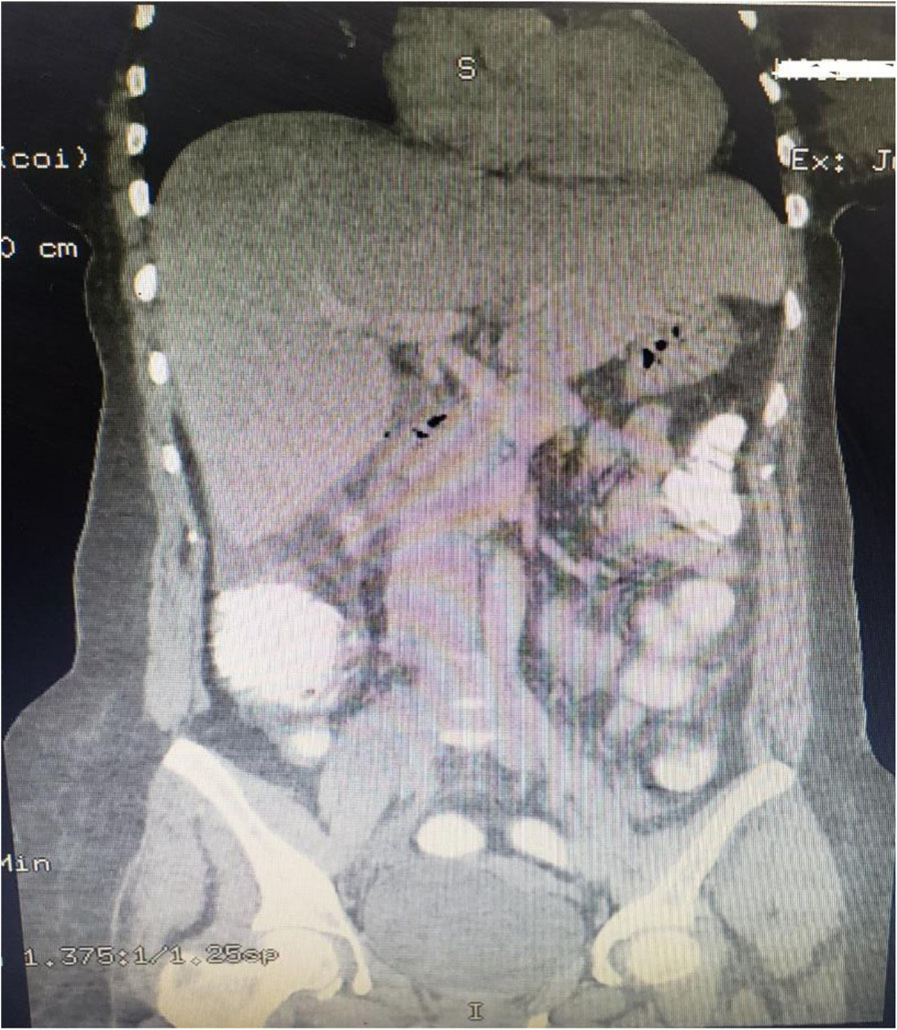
Computed tomographic images showing perforated subhepatic appendicitis with a fecalith
